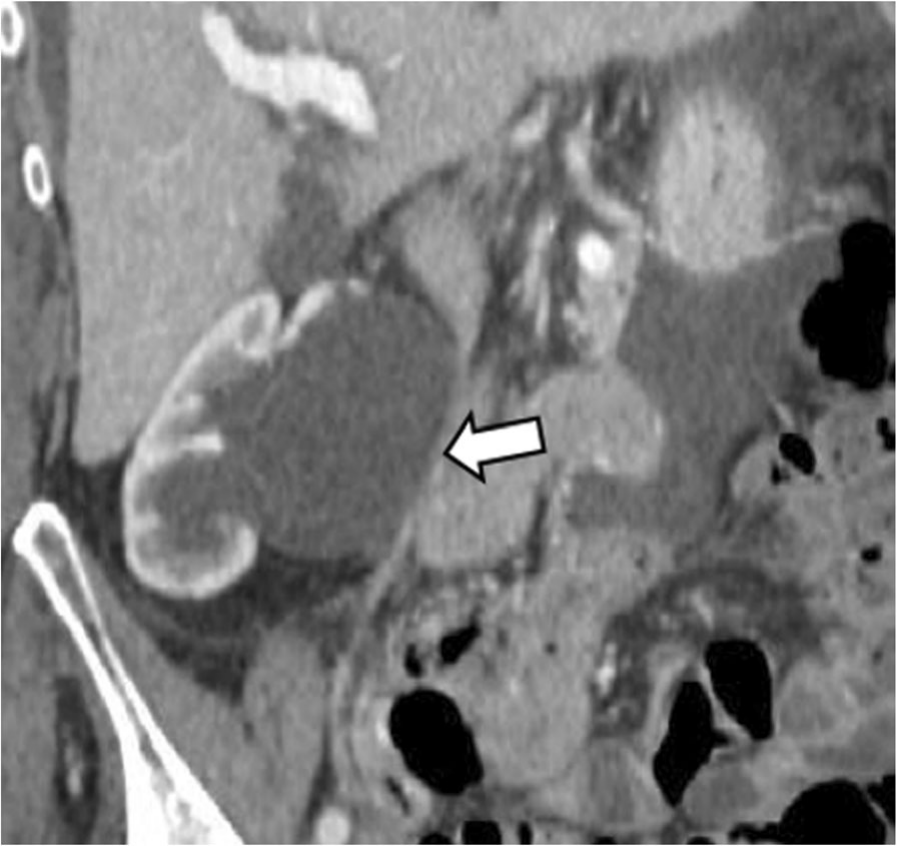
An 84-year-old woman with upper ureteral vascular compression. Coronal enhanced CT image shows the proximal ureter (arrow) compressed by a dilated ovarian vein
